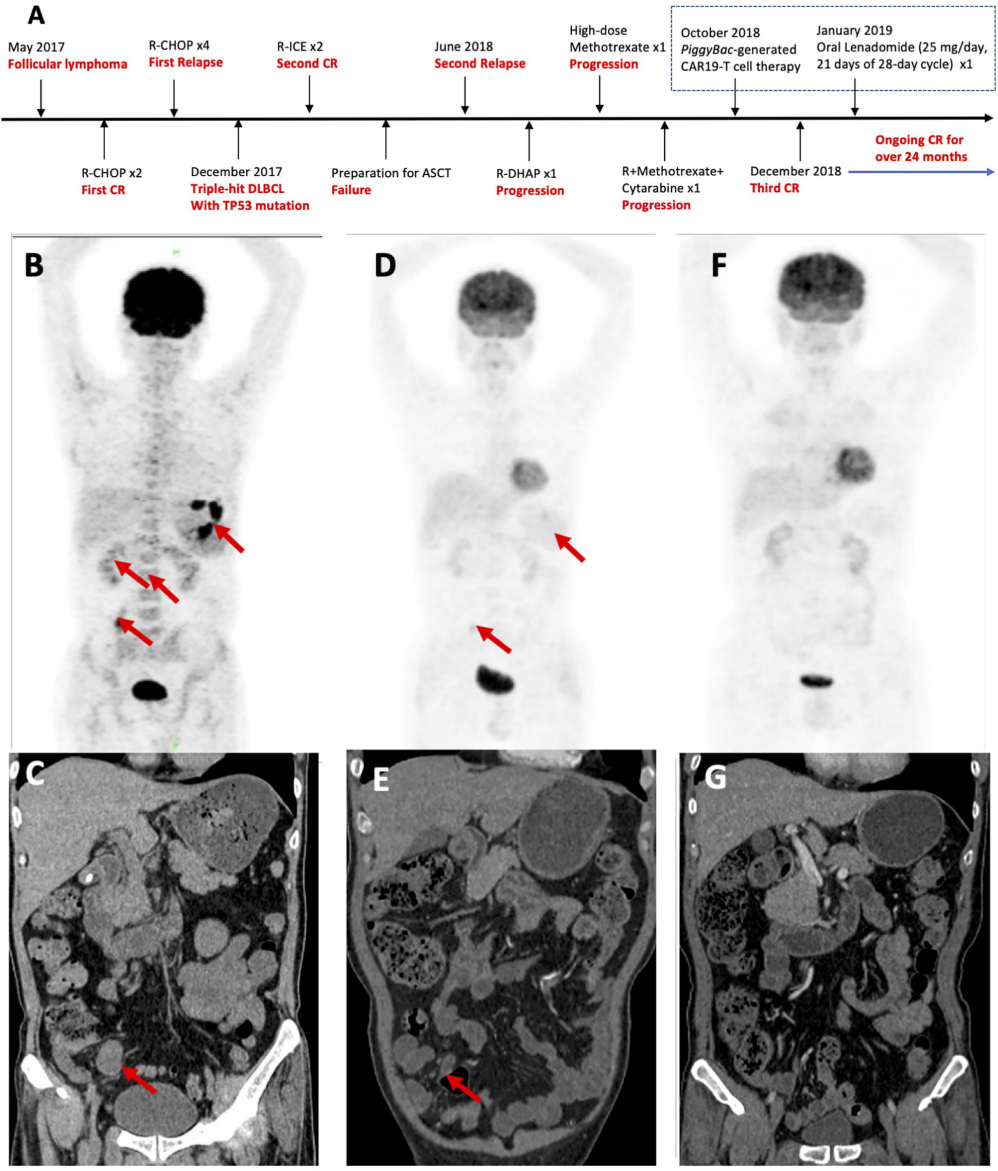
Patient treatment history and response to *piggyBac*-generated CAR19-T cells. (A) Patient’s disease progression and lymphoma treatment history. (B) Pre-treatment PET-CT, showing extensive invasion of tumors in gastric wall (SUVmax 13.2–18.0), small intestines (SUVmax 6.7) and bone marrow (SUVmax 2.6–3.2), scoring 5 points per Deauville criteria. (C) Pre-treatment abdominal CT, showing an abnormal mass sized as 3.0 × 2.2 cm (arrow). (D) PET-CT in the 1st month post-infusion, showing diminished invasion of tumors in small intestines (SUVmax 4.6) scoring 3 points per Deauville criteria. (E) Abdominal CT scans in the 1st month, showing an abnormal mass sized as 1.6 × 1.0 cm (arrow). (F) PET-CT scans were consistent with a complete metabolic response in the 2nd month post-infusion. (G) Normal Abdominal CT in the 2nd month.
